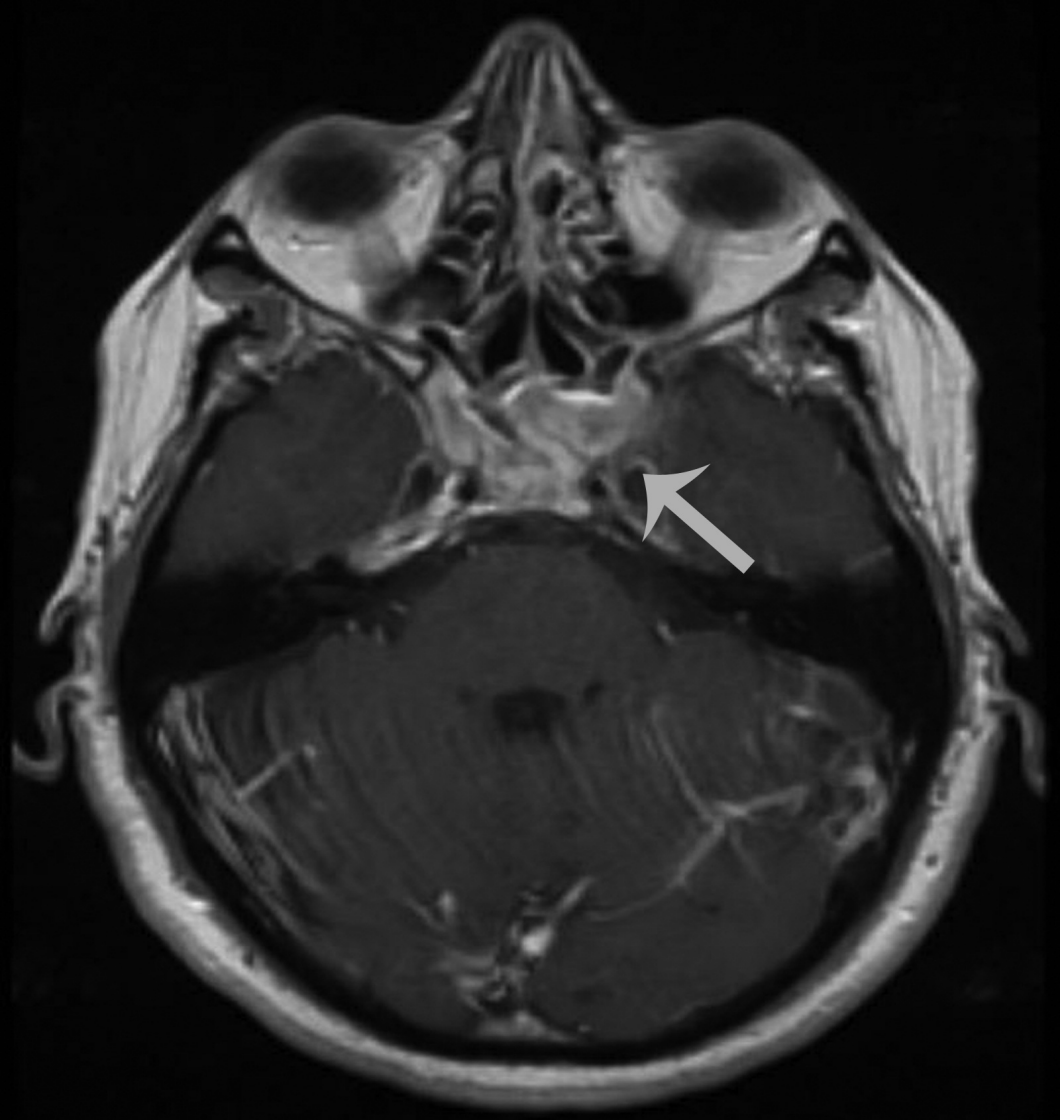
Post-Treatment MRI Axial T1-weighted, contrast-enhanced image showing the same lesion as Figure 3.
